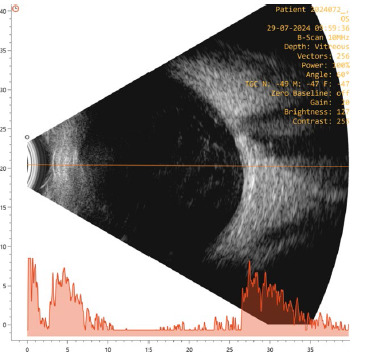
Right eye AB-scan ultrasonography showing dot-like echoes in the vitreous indicative of inflammation, with an attached retina on the day of presentation
